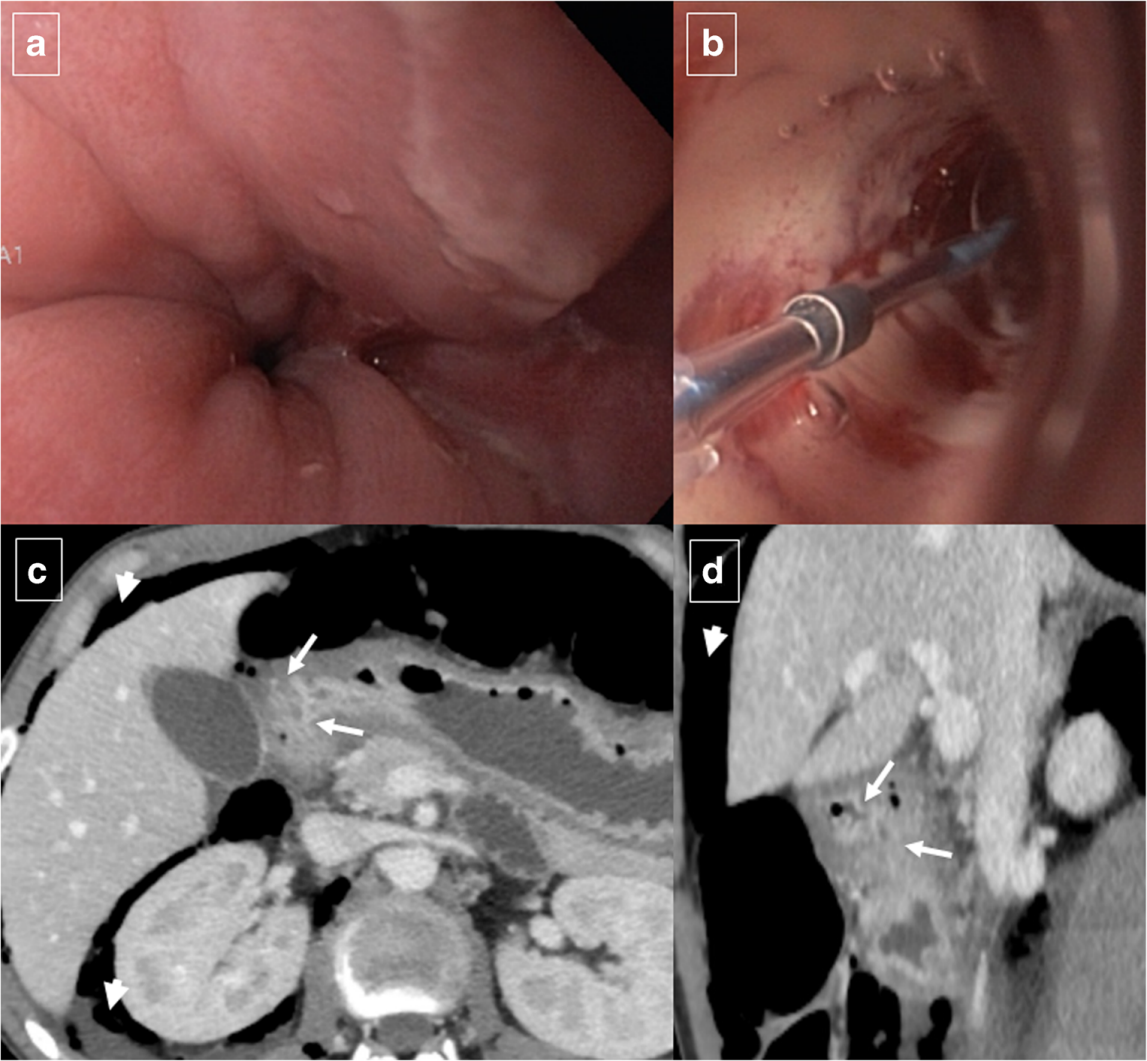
A 24-year-old man with Crohn’s disease who presented with reflux esophagitis, daily vomiting, and food intolerance. a Upper endoscopy shows pyloric and bulbar involvement with ulcerated areas and folded hypertrophy that caused stenosis. After the dilation of the stenosis b the patient presented acute abdominal pain. Axial c and reformatted sagittal d contrast-enhanced CT images confirm the suspected perforation (short arrows) and also show the thickened and hyperaemic wall of the duodenum (arrows) with fibrotic bulbar stenosis
